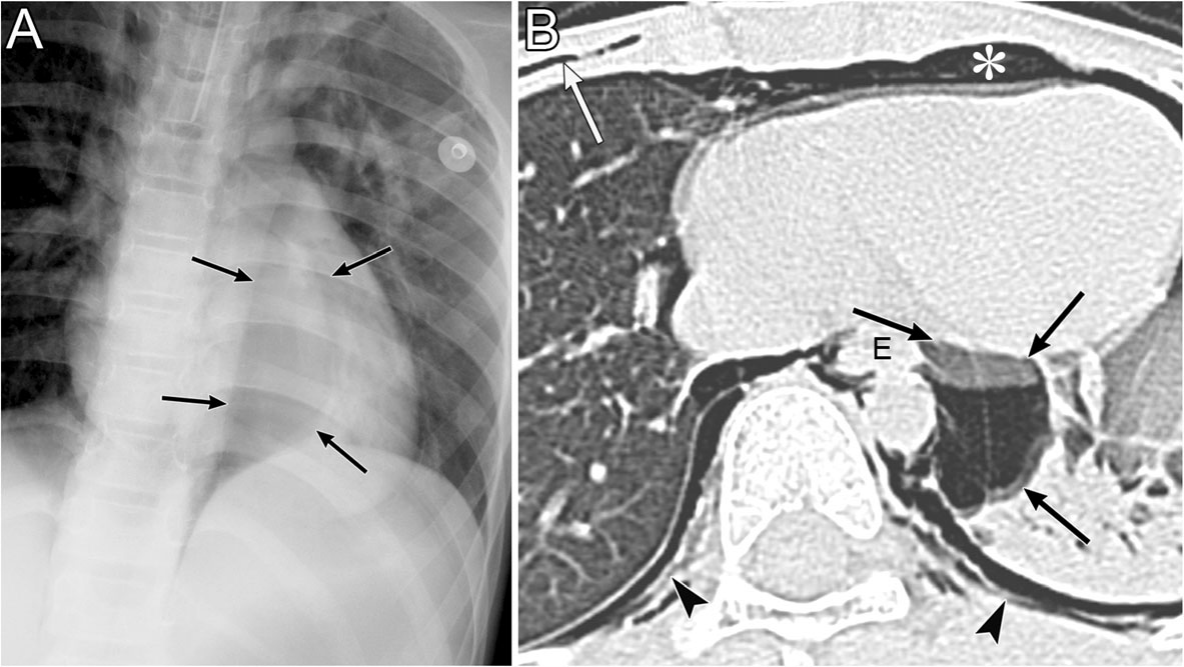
Air collection in the inferior pulmonary ligament (IPL) of an 8-year-old boy with traumatic tracheal injury. a A supine radiograph shows an oval lucency (black arrows) in the left paramediastinal area, which reflects air collection in the left IPL. b An axial CT image reveals air collection in left IPL (black arrows) because air collects along the mediastinum at the level of the esophagus (E). Pneumomediastinum (white asterisk), intrathoracic extrapleural space air collection (black arrowheads), and subcutaneous emphysema (white arrow) are also evident
